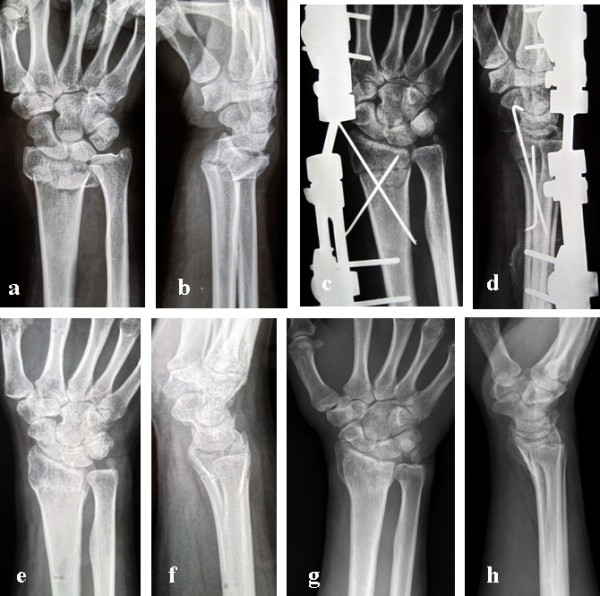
The 65-year-old male patient with unstable distal radius fracture. (a, b) The initial anterior-posterior and lateral radiographs of the distal radius showed an unstable fracture accompanied with the ulnar styloid base fracture: dorsal angulation at 30°; shortening of radius 7 mm. (c, d) The radiographs at the external fixator removal time: the fracture was treated with external fixation augmented with percutaneous Kirschner wires. (e, f) The radiographs at three months postoperatively: the distal radius fracture had united but the ulnar styloid base fracture had not. (g, h) The radiographs at the final follow-up visit: the ulnas styloid base fracture still had not united. Written informed consent was obtained from the patient to show the information here.
